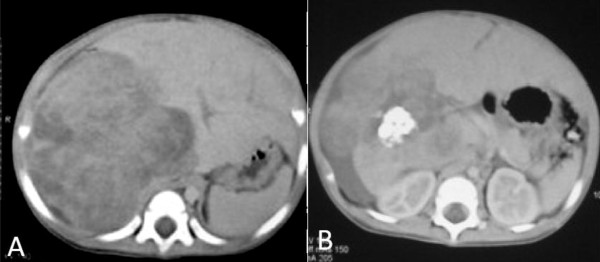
CT scan of the liver showing the hepatic tumor. (A) Involvement of the right hepatic lobe; (B) area calcification and IVC extension.
